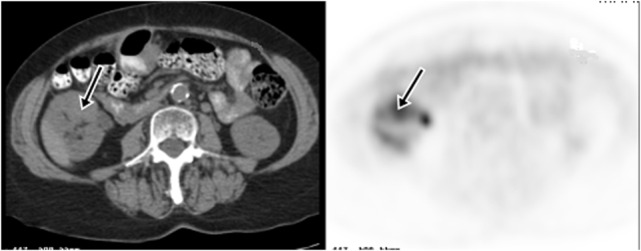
Demonstration of RCC recurrence on FDG PET/CT. A 66-year-old woman had right partial nephrectomy for RCC. Two years later, a diagnostic CT showed a new mass in the anterior midpole of the right kidney, which was FDG avid on PET imaging. Subsequent nephrectomy confirmed recurrence of RCC.
